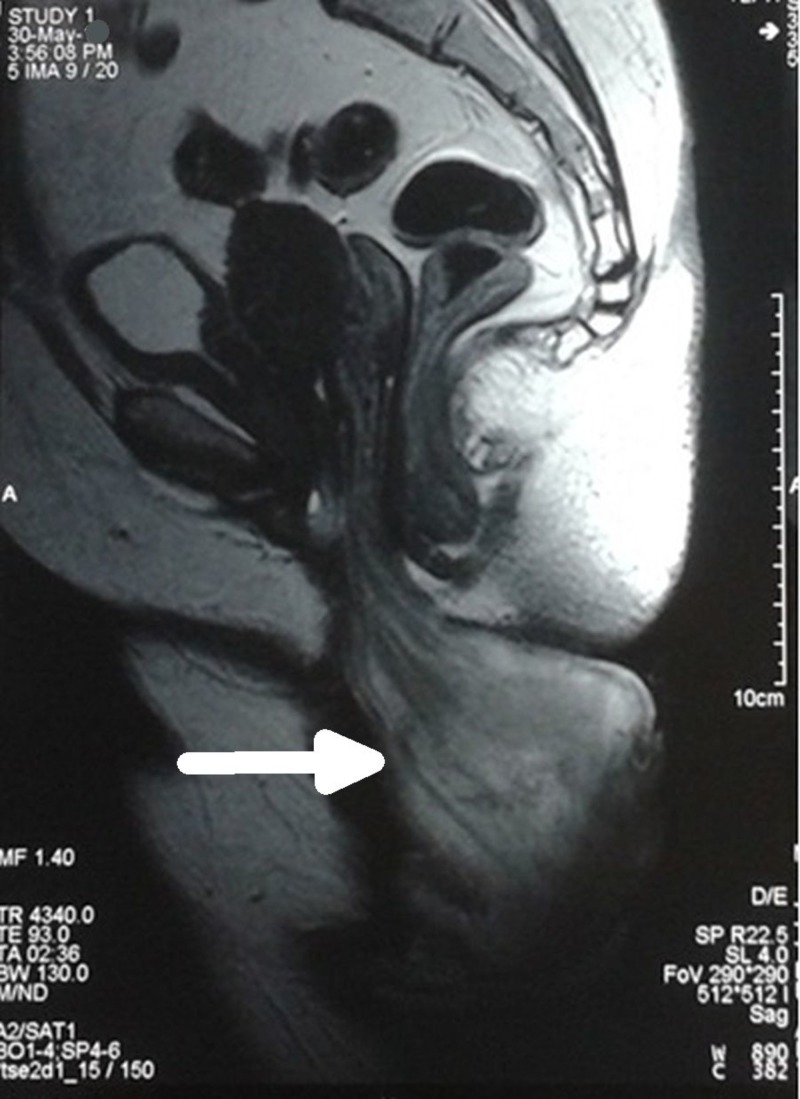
MRI image (Case 1) T2 weighted MRI image of the mass (sagittal view) showing prolapsed mass arising from the cervix and hanging outside vagina (white arrow)
